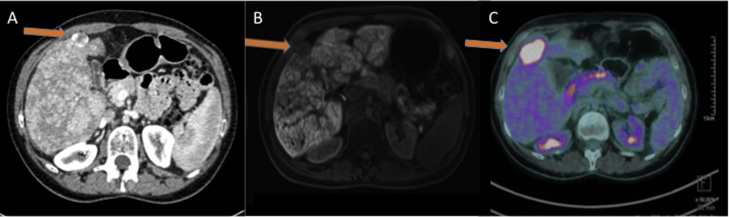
Imaging of hepatocellular carcinoma after Fontan procedure. Pre-operative images in a 33-years old girl with hepatocellular carcinoma and chronic liver disease after Fontan procedure. A) Computer tomography scan showing hypervascular lesion in segment V on arterial phase; B) Axial fat-suppressed T_1_-weighted Magnetic resonance imaging with gadoxetic acid administration obtained at 20 min, hepatocellular phase, detecting strong enhancement of the background liver parenchyma, but no uptake in the hepatocellular carcinoma; C) Fluorine-18 fluorodeoxyglucose (FDG) positron emission tomography showing increased FDG uptake in the hepatic lesion.
